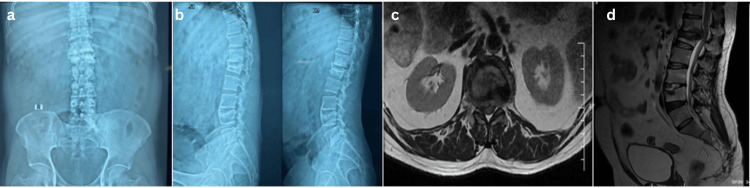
Spinal case 4 (a) Plain X-ray of the lumbar spine in the anteroposterior (AP) view shows the collapse of the L1 vertebrae with paradiscal sclerosis at D12 and the L1 disc level. (b) Plain X-ray of the lumbar spine (flexion and extension) in the lateral view shows compression of L1 with loss of height and no significant movement on dynamic views. (c, d) MRI of the lumbar spine in the axial view and T2 sagittal views, respectively, showing severe T12-L1 disc retropulsion with compression of the conus medullaris and nerve roots, along with reactive inflammatory changes at this level. The data shown are representative radiographic and MRI findings from a single patient case (spinal case 4). Original images obtained by the authors at Subbaiah Institute of Medical Sciences, Shivamogga.
